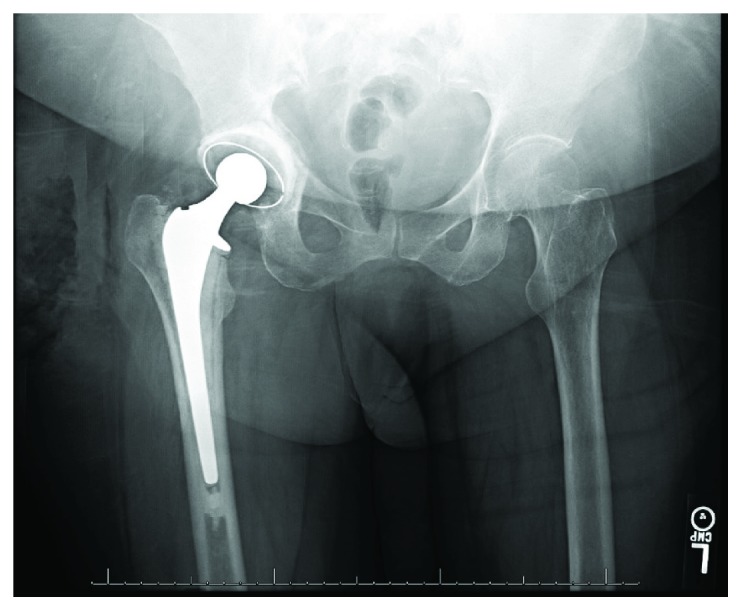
AP pelvis radiograph obtained following right revision total hip arthroplasty.
